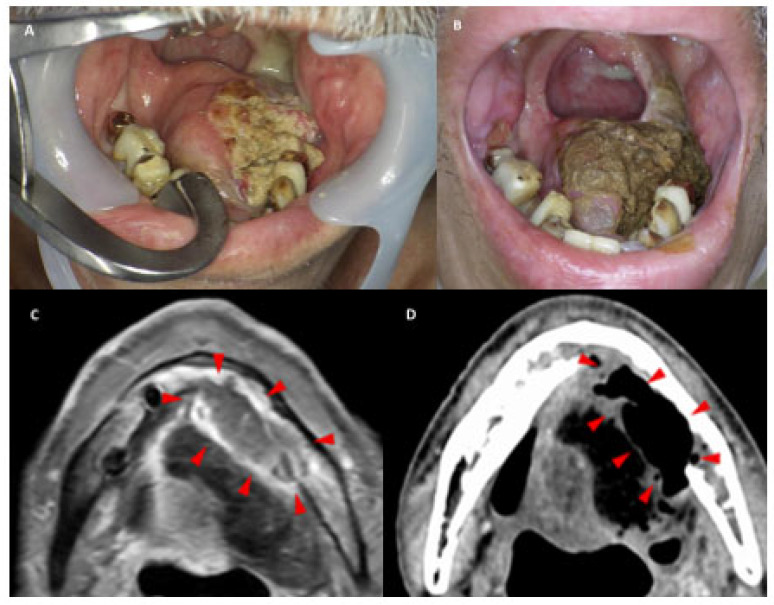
A representative case of recurrence after tongue cancer reconstruction surgery. (A) Tumor recurrence observed on the lateral aspect of the mandible, medial to the reconstructed tongue. (B) Tumor necrosis with marked tumor shrinkage observed 1 month post-treatment. (C) Axial contrast-enhanced T1-weighted MRI showing the extent of the tumor (arrowheads). (D) Axial contrast-enhanced CT scan obtained 1 month post-treatment shows a marked reduction in the tumor (arrowheads). MRI, magnetic resonance imaging; CT, computed tomography.
